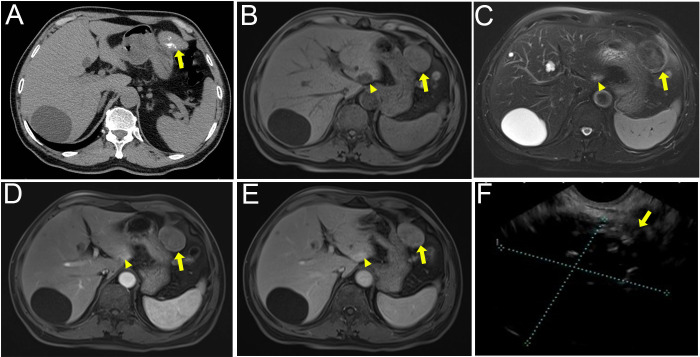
Imaging findings of the gastric IMT and hepatic hemangioma. (A) CT showing a heterogeneous mixed-density mass with focal calcifications. (B, C) T1- and T2-weighted MRI showing low signal intensity of the gastric mass. (D, E) Arterial- and venous-phase contrast-enhanced MRI showing moderate enhancement of the mass. (F) EUS showing a well-demarcated hypoechoic-to-anechoic lesion arising from the muscularis propria with an exophytic growth pattern. Arrows indicate the GIMT, and arrowheads indicate the hepatic hemangioma.
